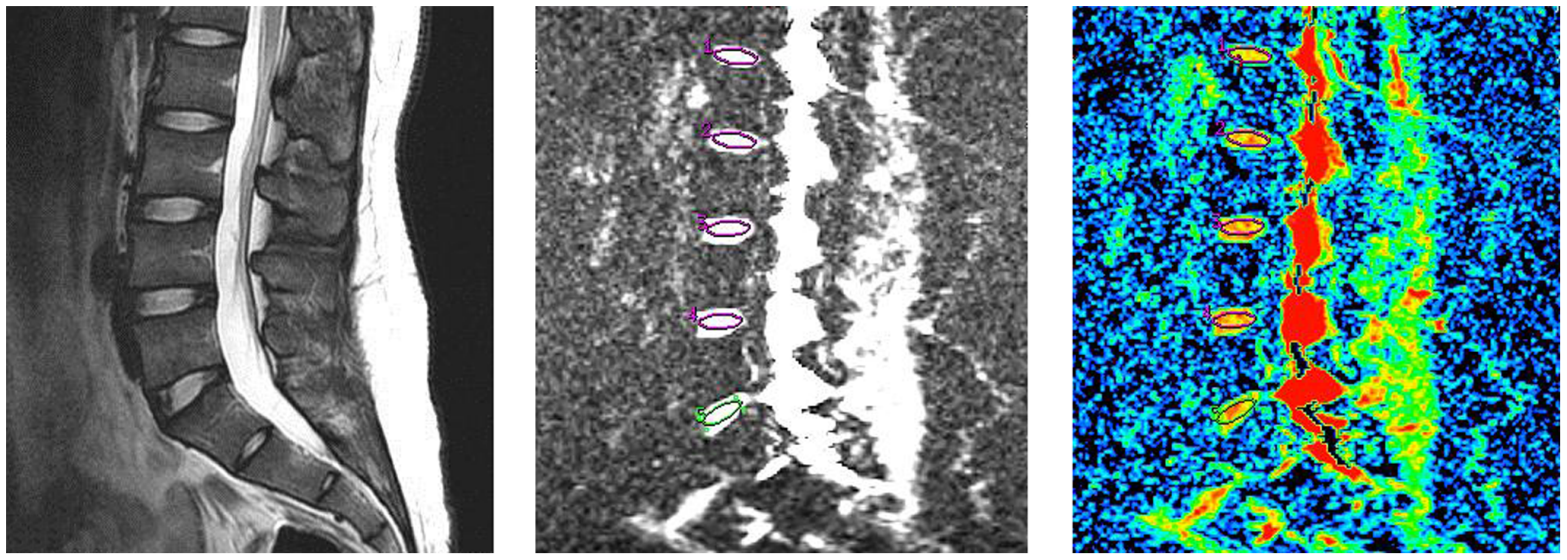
T2, DWI and ADC images of the lumbar intervertebral discs in a 26 years participant. The T2 intensity of the subject from L1–2 to L5-S1 were 92.6, 92.3, 95.1, 97.0, 95.8ms and the respective ADC values were 168, 178, 184, 182, 187×10^−5^ mm^2^/s.
